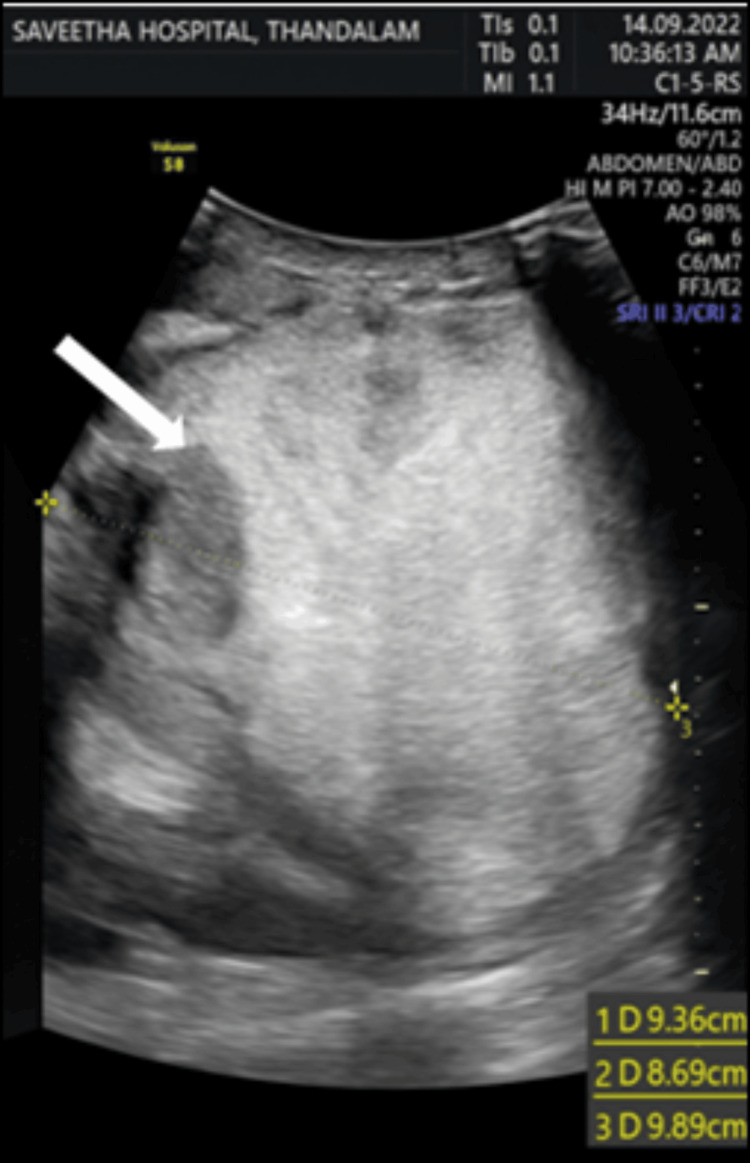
Scrotal ultrasound showed a large heteroechoic solid mass (white arrow) measuring approximately 9.9 x 9.4 x 8.7 cm, with hyperechoic areas seen expanding the right hemi-scrotal sac. Both testicles were seen separately; however, the testicular origin of the mass lesion could not be confirmed, necessitating cross-sectional imaging.
